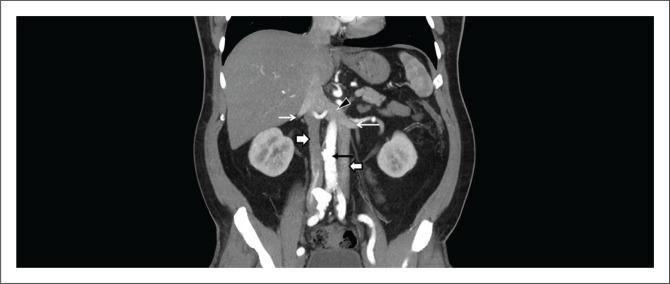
A 31-year-old female with acute right lower quadrant pain related to a haemorrhagic cyst. Contrast enhanced coronal CT image demonstrated duplication of the inferior vena cava (IVC) (white thick arrows) with the aorta (black thin arrow) in between. The renal veins (white thin arrows) are draining into the ipsilateral IVC. The left IVC after joining the left renal vein courses to the right to communicate (black arrowhead) with the right IVC.
